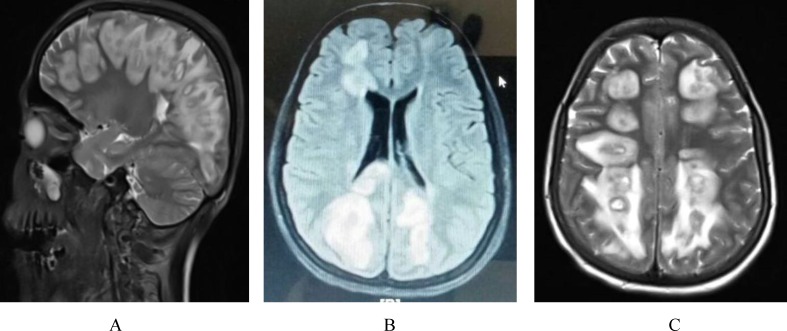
Sagittal T2W. Incidental note is made of maxillary sinusitis. (A), axial fluid-attenuated inversion recovery (FLAIR) Right aspect of corpus callusum (posterior part of body splenium junction) involvement (B), and axial T2W (C). Brain MRI images demonstrate the mild asymmetrical diffuse and dispread bilateral white matter abnormal high signal intensity at periventricular and centrum semiovale area
